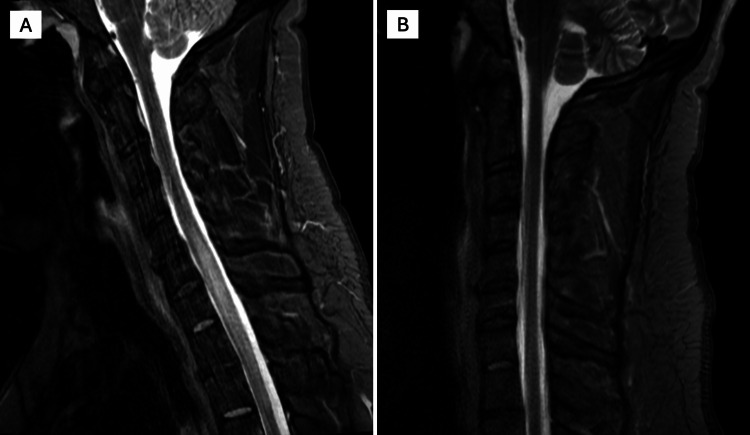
Spinal lesion of neurosarcoidosis (A) MRI of the cervical spine showing T2 hyperintensity in the cervical spinal cord from C5-T2; (B) Repeat cervical MRI at six-month follow-up showing resolution of hyperintensity.
